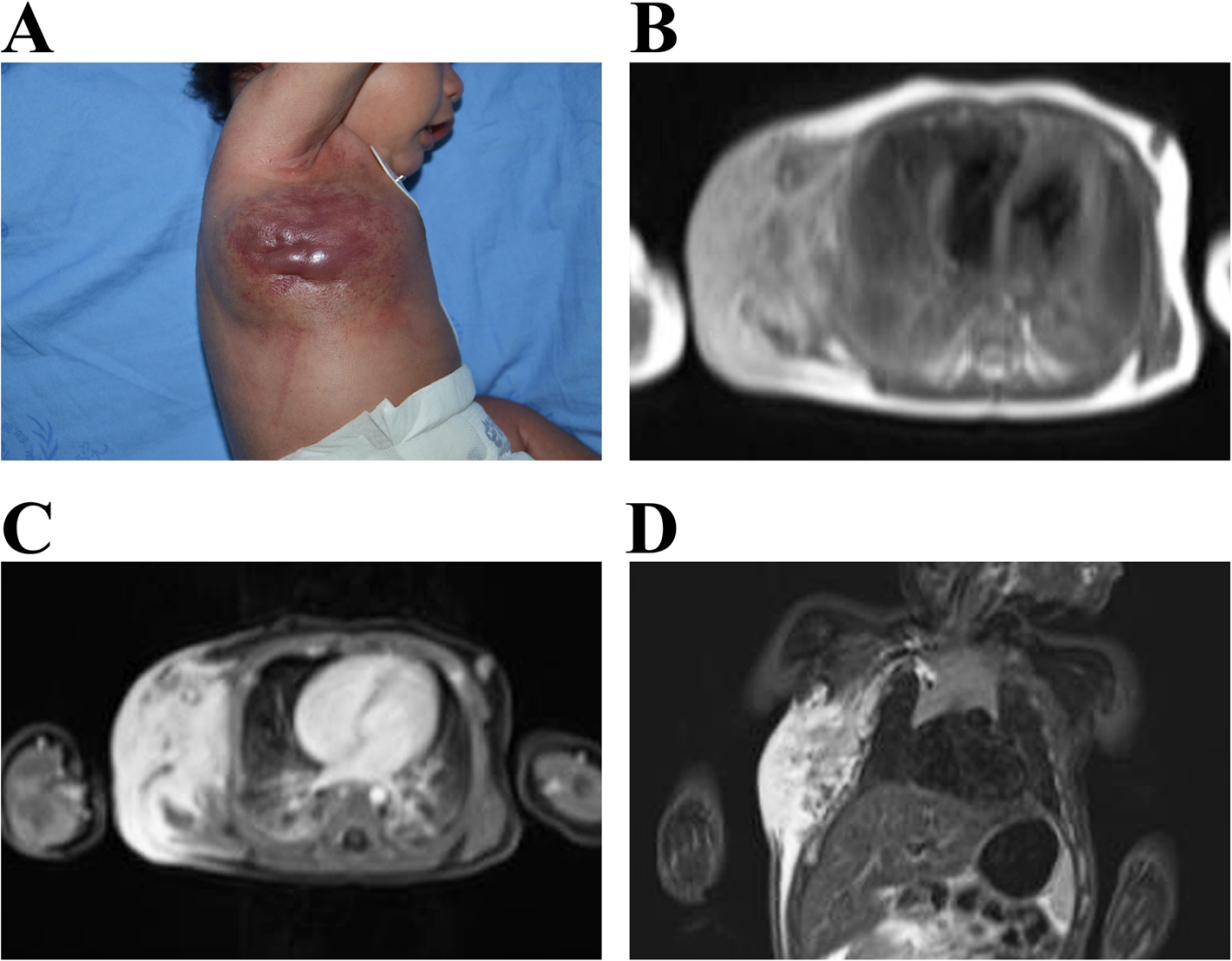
Clinical and MRI features of KHE with KMP. a A 2-month-old boy was found to have a chest wall mass after birth. The mass became progressively indurated and purpuric. The boy developed profound thrombocytopenia and consumptive coagulopathy. b Horizontal T1-weighted MRI revealed that the heterogeneous mass was isointense relative to the adjacent muscle on T1-weighted imaging. Horizontal (c) and sagittal (d) T2-weighted MRI revealed hyperintense lesions infiltrating the right lateral chest wall
